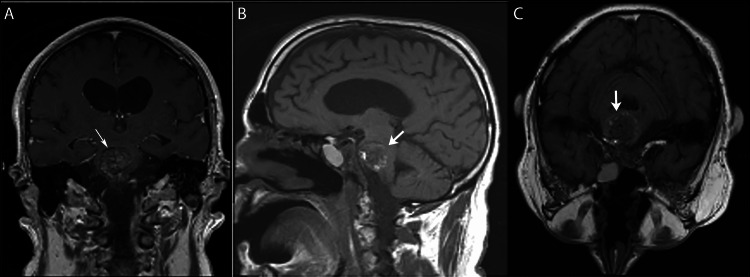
Pre-operative MRI T1 scans in coronal (A), sagittal (B), and axial (C) views demonstrate the recurrent lesion. The MRI series shows a T1/T2 heterogeneous enhancing lesion in the pons with associated vasogenic edema, initially thought to be secondary to hemorrhagic cavernoma. A heterogeneously enhancing clival lesion extends into the retroclival and prevertebral regions, encasing the basilar artery, suggesting residual/recurrent chordoma.
